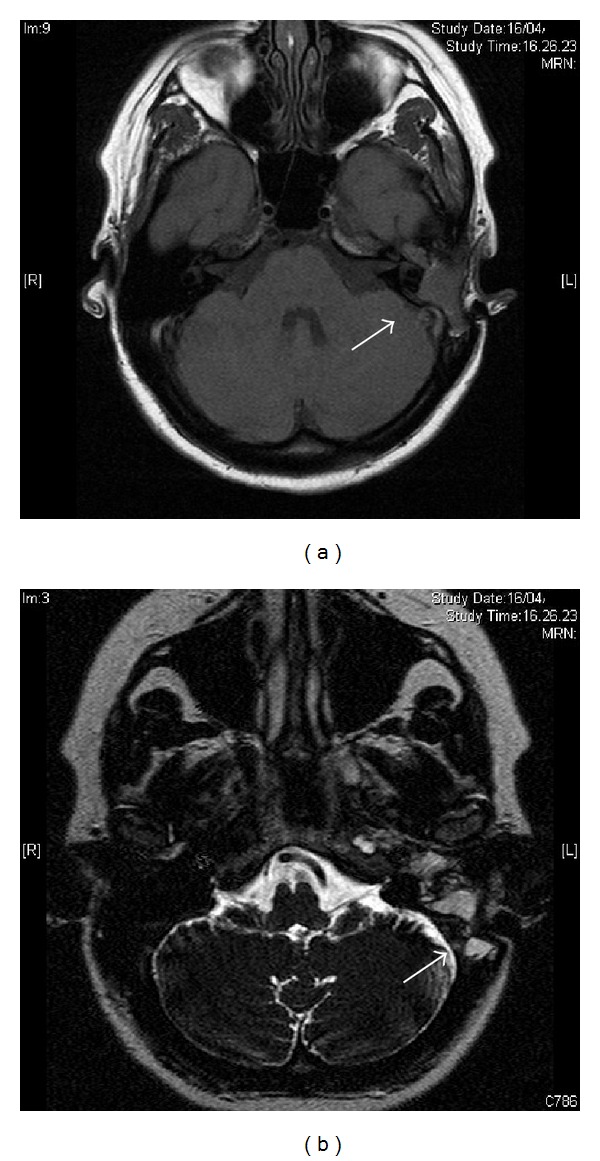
Axial T1-weighted cerebral MRI confirmed the presence of a lesion of increased signal, similar to brain tissue (a); the tumor (22 × 15 × 11 mm) enhanced with gadolinium in the medial portion of middle ear and in the tubaric region (b).
